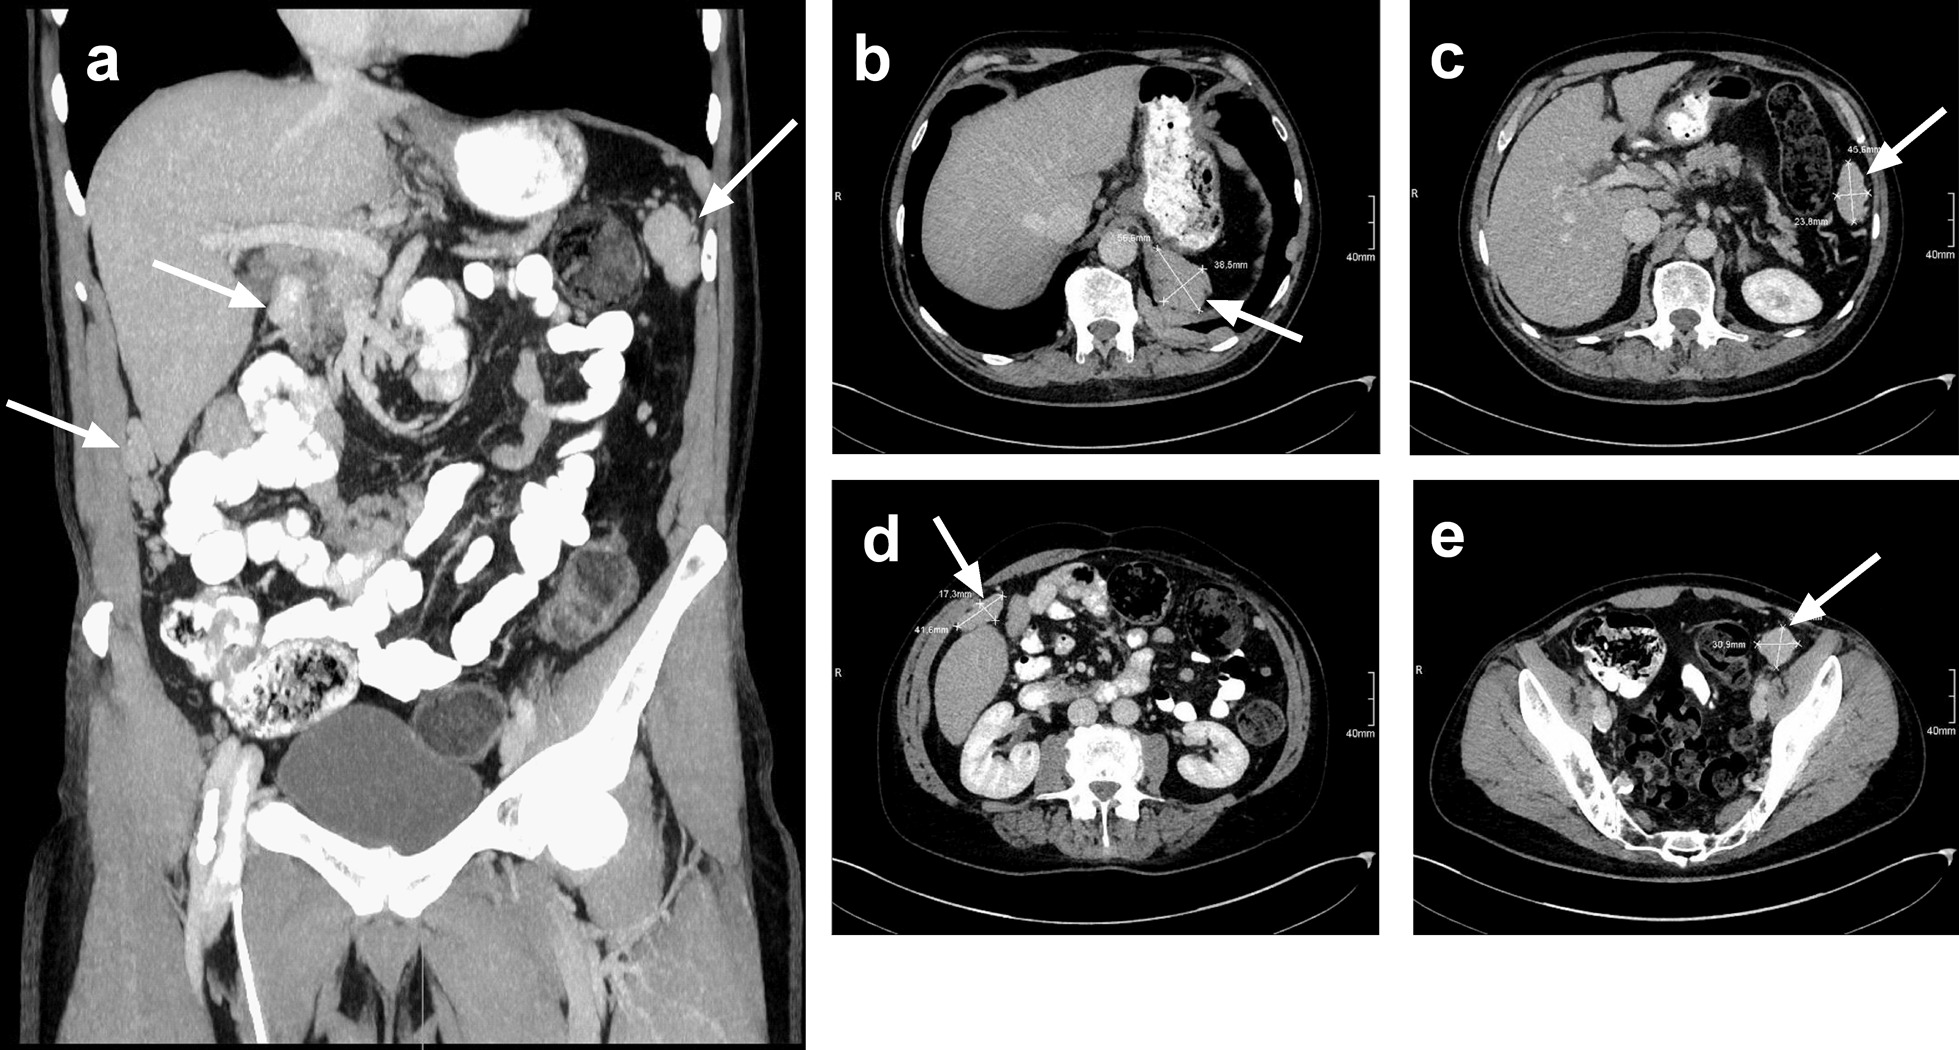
Contrast-enhanced abdominal CT scan (portal phase). Coronal (a) and axial planes (b–e) of the abdominal and pelvic cavities. White arrows indicate masses under the left diaphragm (b, c), between the abdominal wall and liver (d), and in the pelvic cavity (e)
